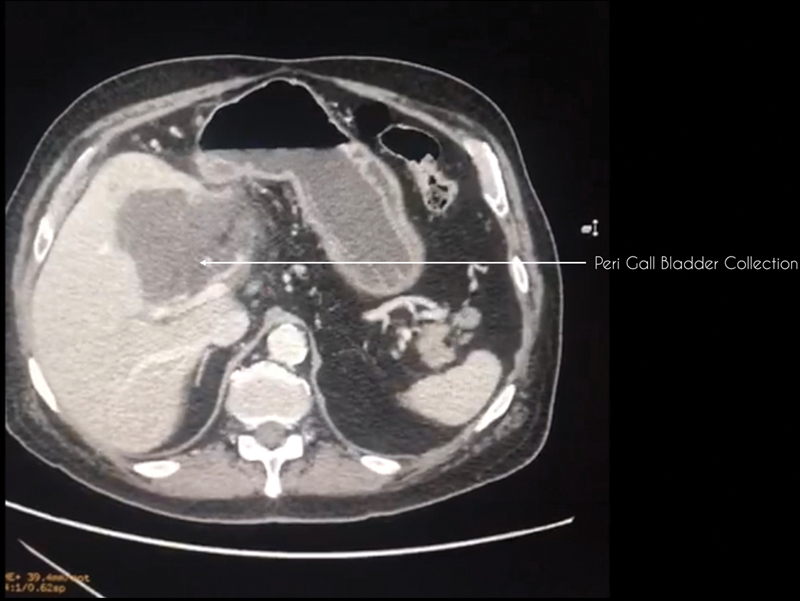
CT image showing collection in the gall bladder fossa suggestive of a perforated gall bladder. CT, computed tomography.
